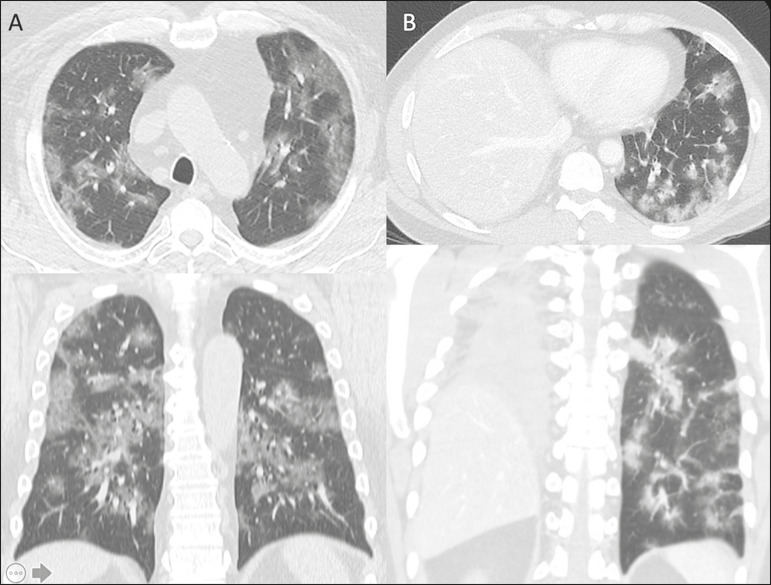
Examples of typical findings for COVID-19 on chest CT. A: Multifocal bilateral ground-glass opacities with typical distribution. B: Multifocal ground-glass opacities in a peripheral and lower distribution in the left lung in a patient with prior right pneumonectomy for lung cancer. Both cases had positive RT-PCR test for SARS-CoV-2 (true-positive results).
